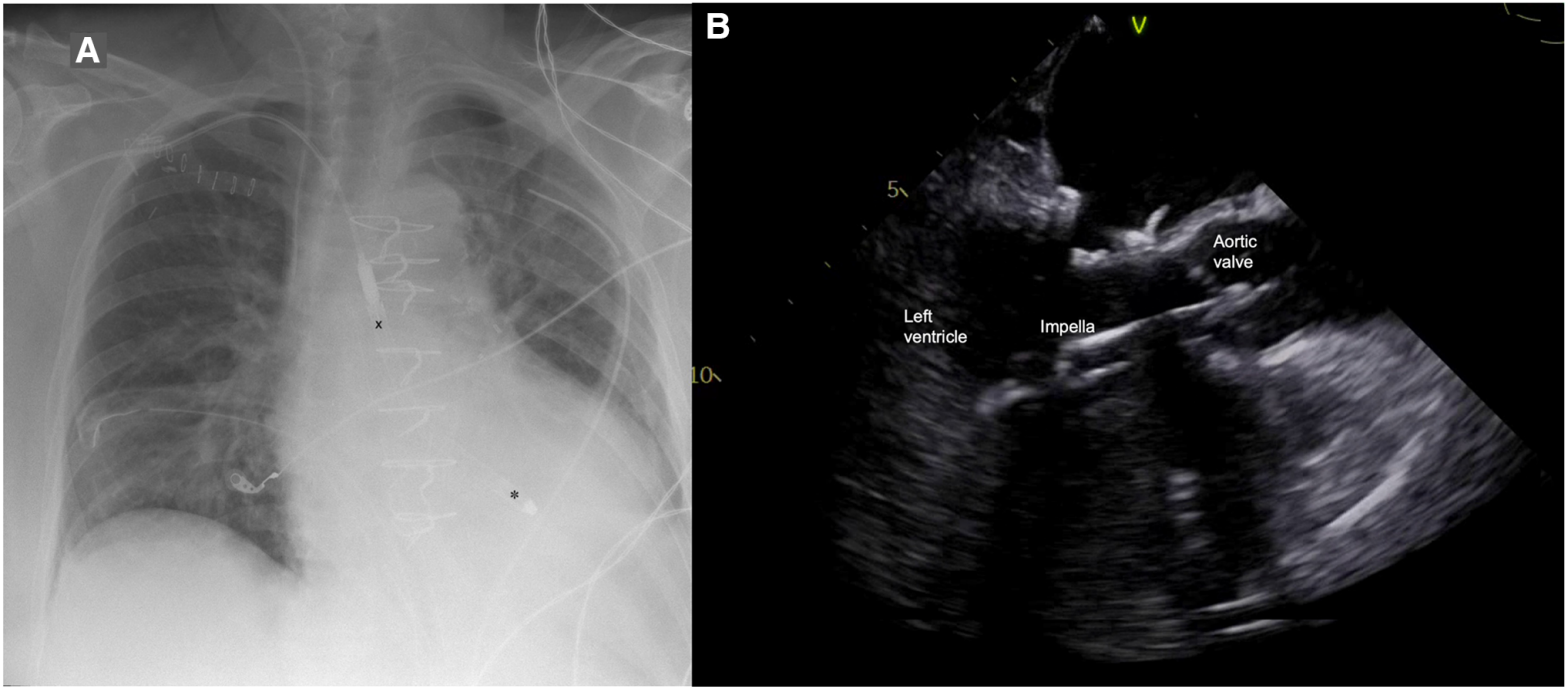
(A) Postoperative chest x-ray with Impella 5.5 pump (X outflow, ✽ inflow); (B) Impella position in transesophageal echocardiography.
